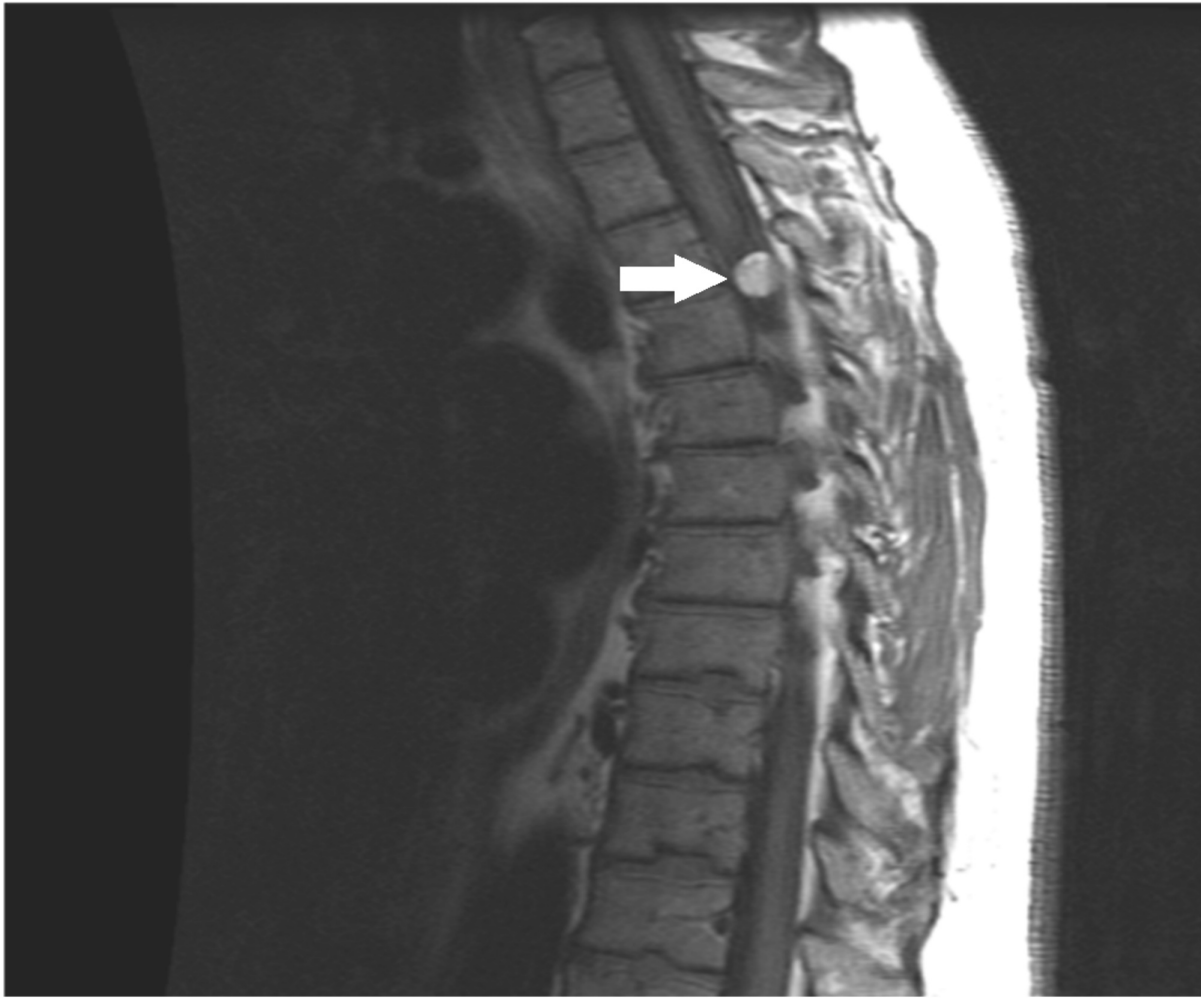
Preoperative T1 sagittal MRI showing a hyperintense lesion at the T3-T4 level MRI: magnetic resonance imaging
